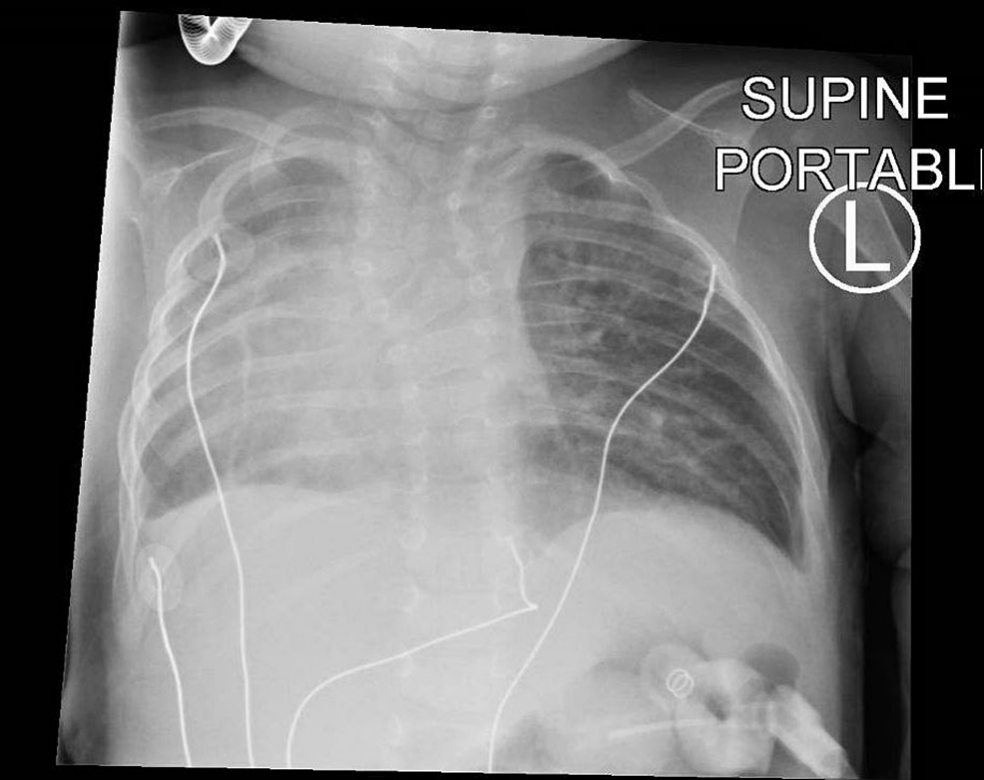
Initial chest X-ray demonstrating right pulmonary hypoplasia, dextroposition, and scoliosis
